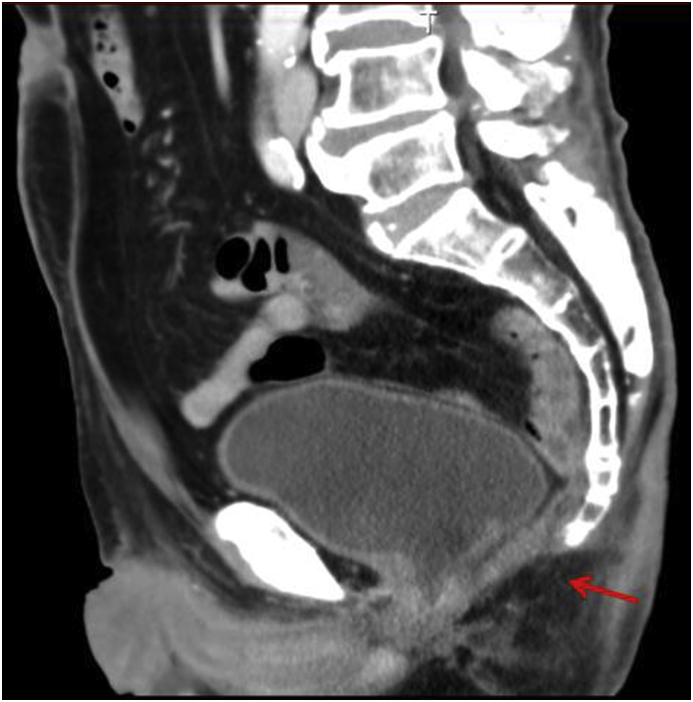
The post-operative CT scan showed that the levator ani was closed with prostate suture (arrow).
